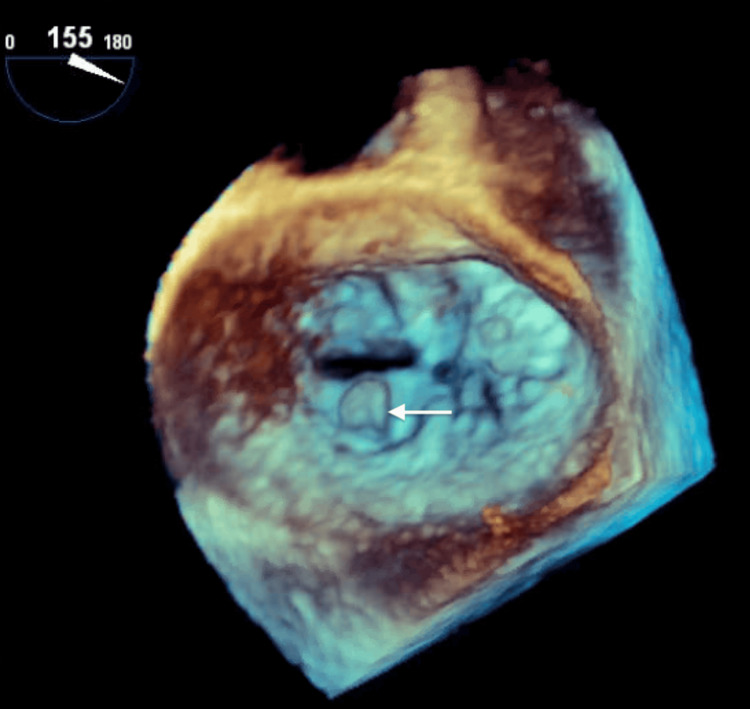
Three-dimensional echocardiogram demonstrating a mass on the mitral valve. The white arrow shows mass on the mitral valve.
